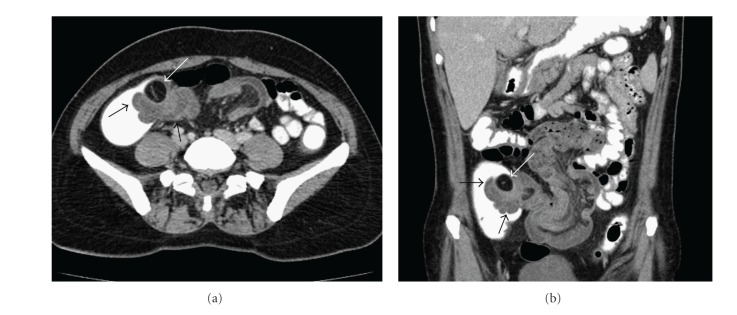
(a) Axial and (b) coronal plan intravenous contrast-enhanced CT scans with oral and rectal contrast demonstrate an ileocolic intussusception with diffuse wall thickening of terminal ileum and the entrance of ileal segment into the cecum at the ileocecal valve level (black arrows). In the cecum adjacent to invaginated segment, hypodense mass lesion consistent with lipoma is seen (white arrow).
